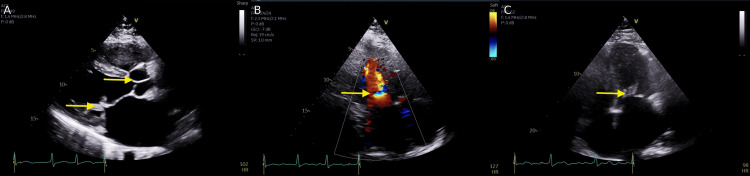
Parasternal long axis view of echocardiogram showing stenotic orifice of mitral valve (A). Apical 4-chamber view showing regurgitant jet on colour Doppler (B) and stenotic mitral valve on similar view (C) Findings labelled by yellow arrow
